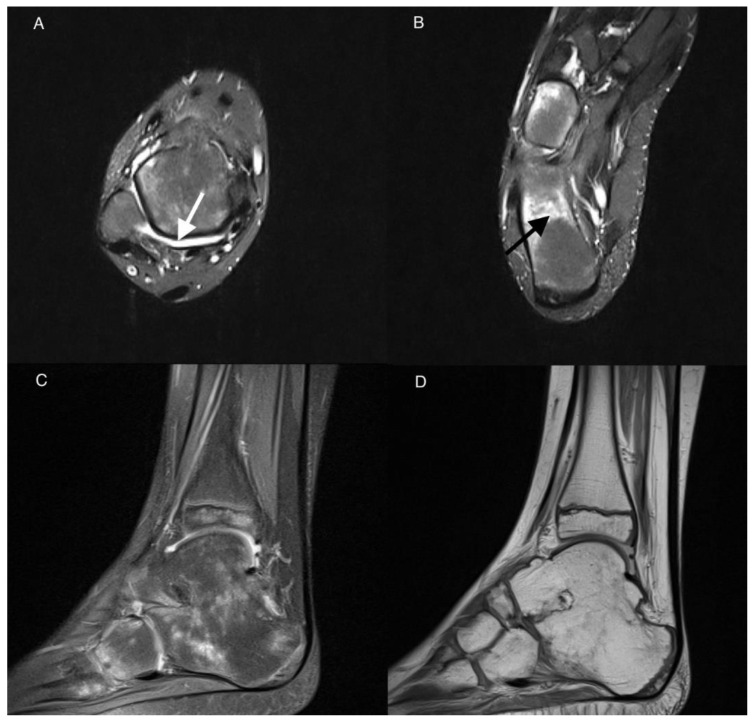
MRI of the right ankle of a 9-year-old boy with JIA. Axial T2-weighted TIRM images (A,B), sagittal PD with fat saturation (C), and sagittal PD-weighted image (D). Effusion/synovial thickening stage 2 in the tibio-talar joint (arrow). BME in numerous bones of the ankle and tarsum, the most advanced in calcaneus stage 3 (arrow in (B)). Tibio-talar joint space narrowing, tibio-calcaneal ankylosis, and osteophytes (C,D).
